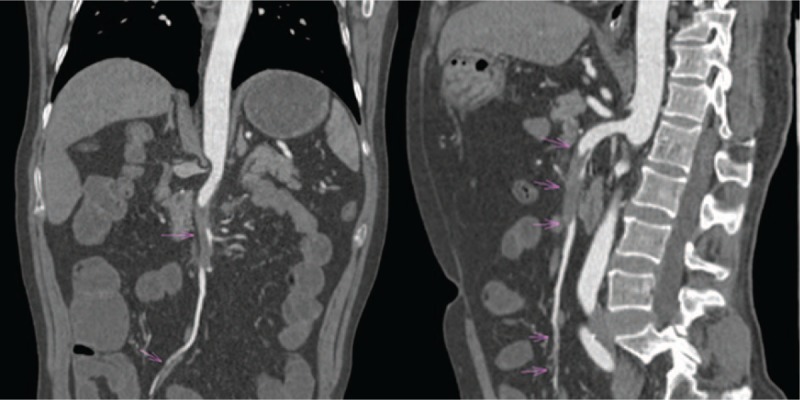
Computed tomography scan of the abdomen showing the area of superior mesenteric artery and ileocolic artery dissection with false lumen thrombosis (arrow).
